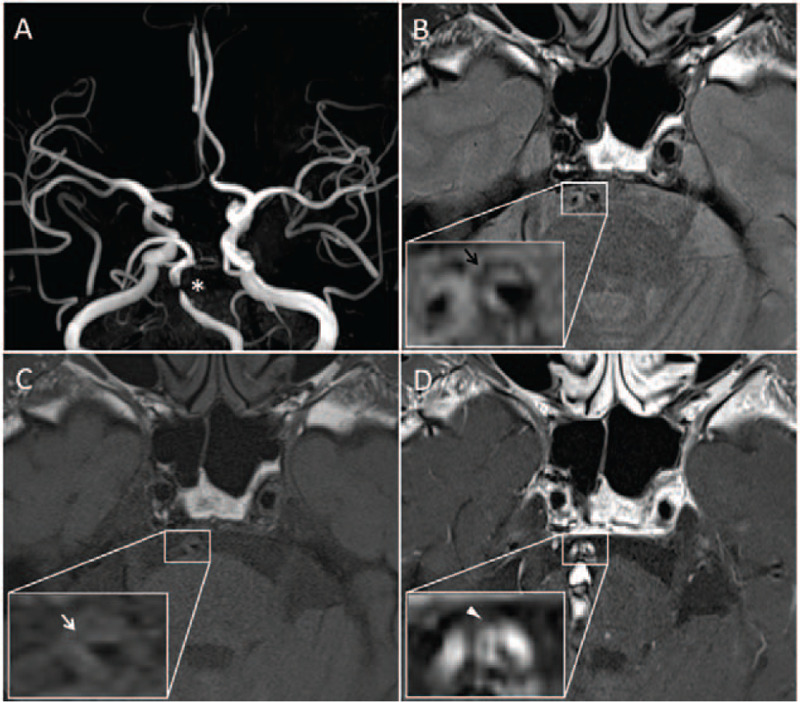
High-resolution magnetic resonance imaging (HRMRI) of our patient. (A) Time-of-flight MR angiography shows severe stenosis in the basilar artery (^∗^) (B) Proton density sequence shows eccentric wall thickening and atherosclerotic plaques (black arrow). (C) T1-weighted image of HRMRI shows eccentric atherosclerotic plaques without intraplaque hemorrhage (white arrow). (D) Contrast-enhanced T1-weighted image of HRMRI shows strong enhancement in eccentric atherosclerotic plaques (white arrowhead). HRMRI = high-resolution MRI, MRI = magnetic resonance imaging.
